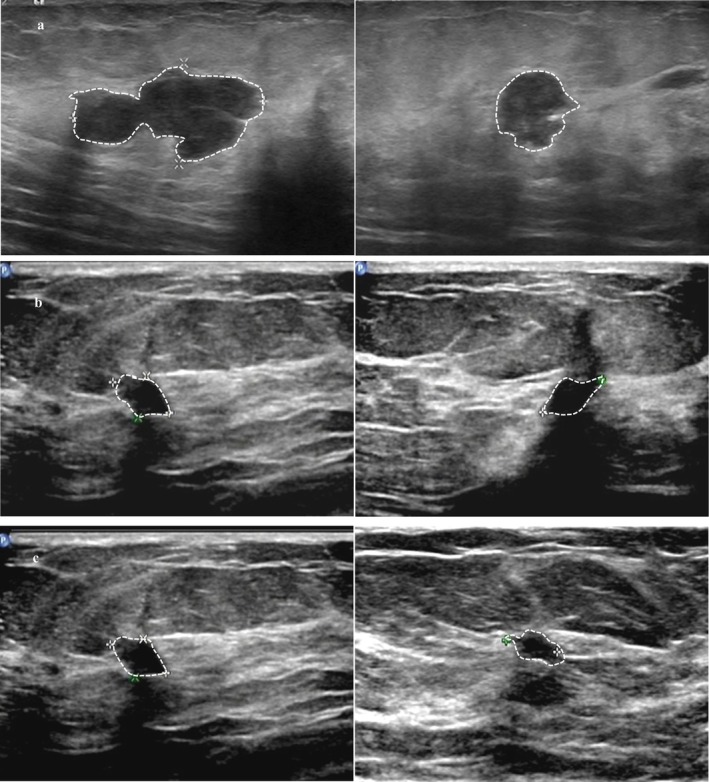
Case 1. A 64‐year‐old female with a left breast mass, classified as BI‐RADS category 5, biopsy confirmed as invasive ductal carcinoma, HER‐2 positive breast cancer (ER 90%, PR 40%, HER‐2 3+), and TILs at 30%. She received six cycles of neoadjuvant therapy. Ultrasound imaging before NAT (a) showed the tumor as hypoechoic. No significant changes in echogenicity were observed during the 2nd (b) and 6th (c) cycles of NAT, with a tumor volume reduction rate of 77% after the second cycle. Pathology after breast‐conserving surgery confirmed a pathological complete response (pCR).
